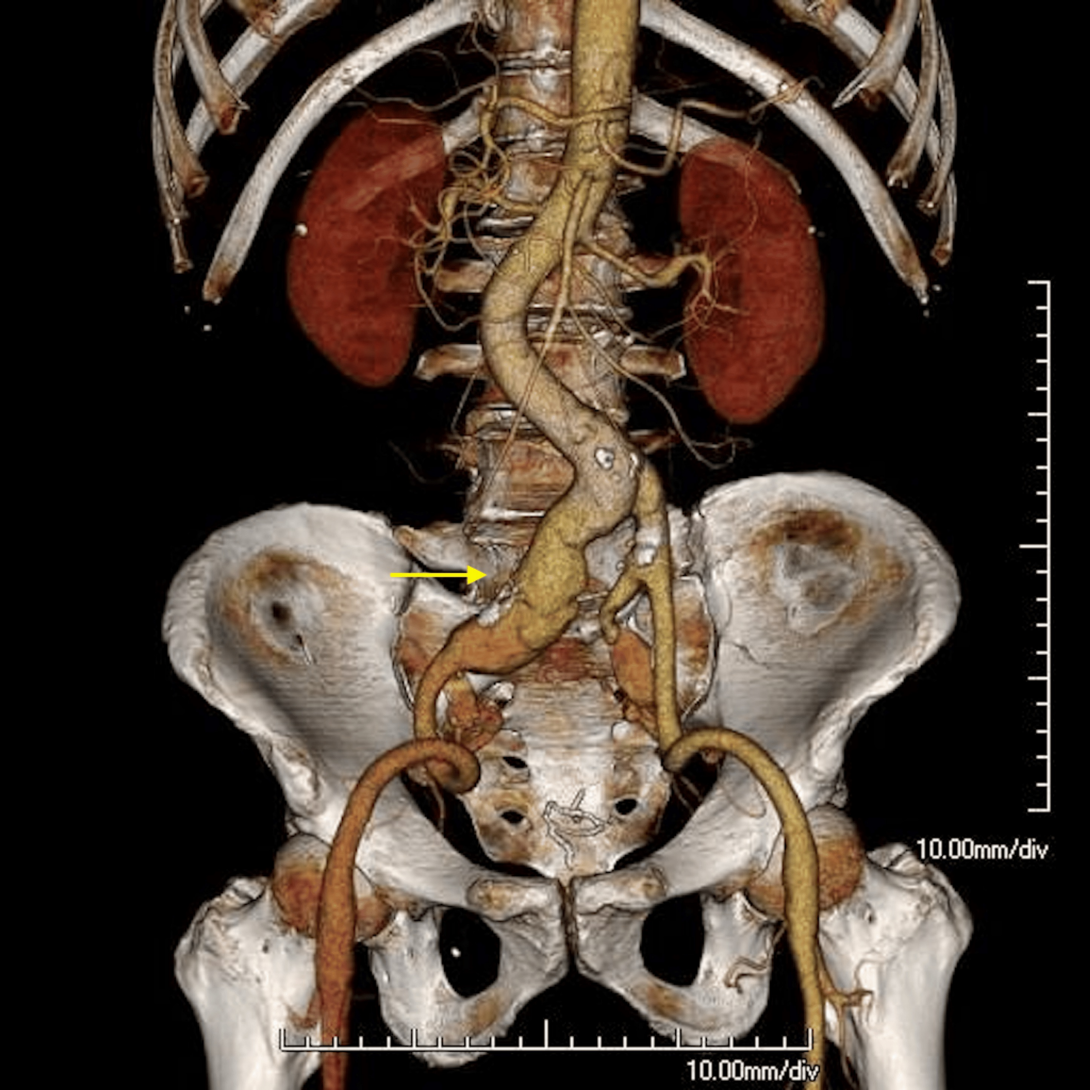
Volume-rendered reconstruction of CTA before EVAR The arrow indicates a right common iliac artery aneurysm measuring 35 mm. CTA, CT angiography; EVAR, endovascular aortic aneurysm repair
